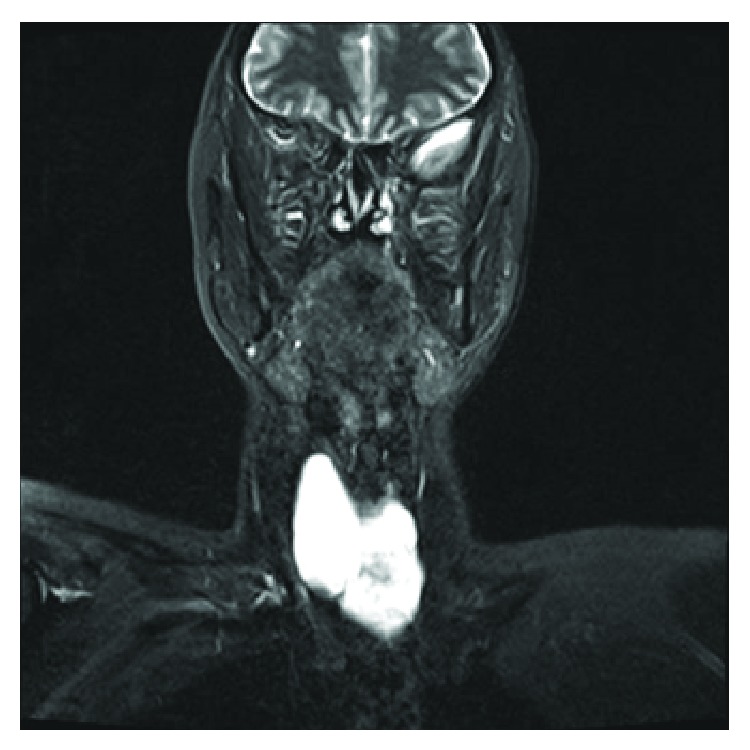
T2-weighted coronal MRI demonstrating a pyramidal shaped cystic lesion in continuity with the lower portions of both thyroid lobes.
